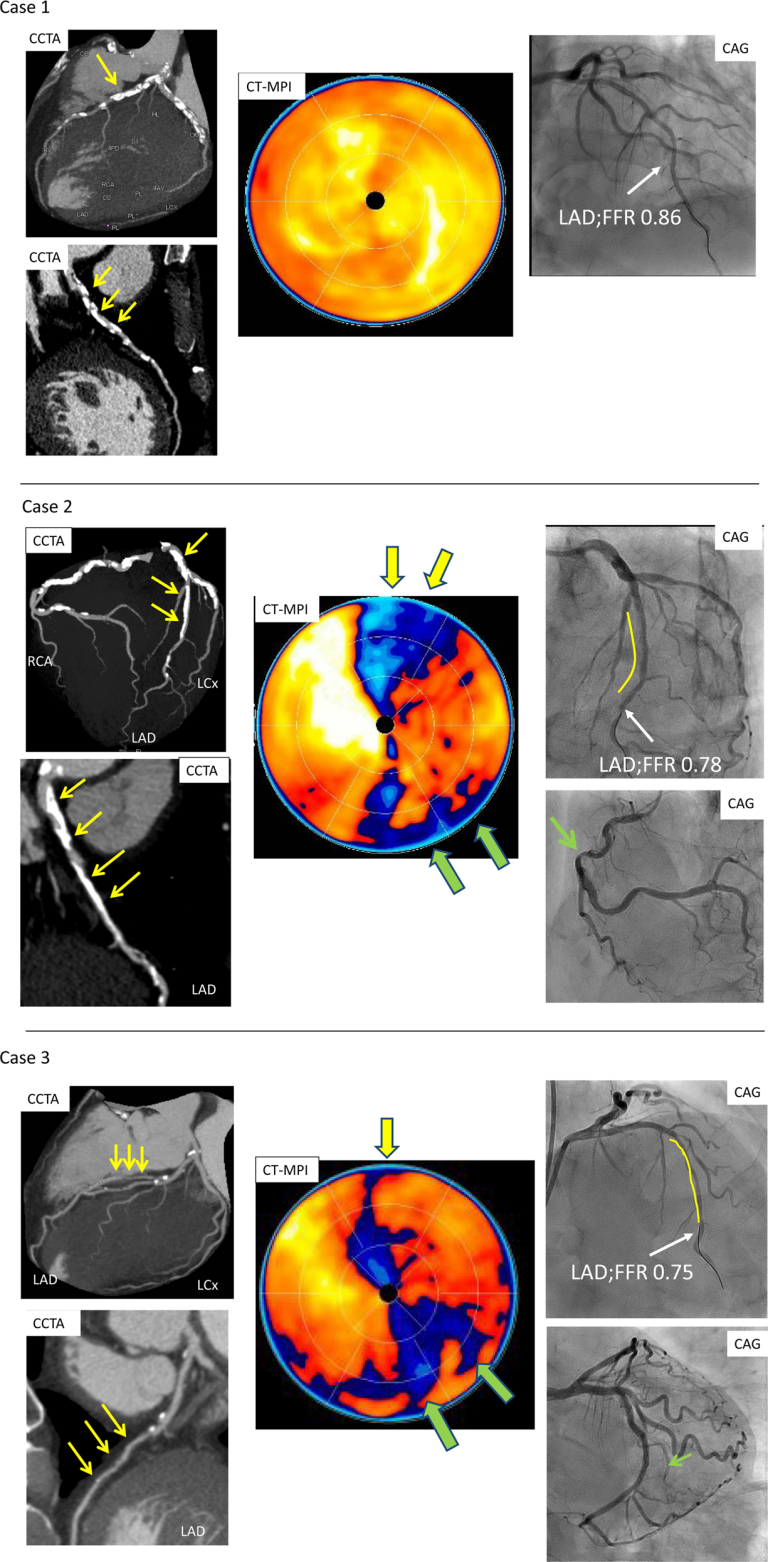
Case examples of CCTA, CT-MPI without stress, coronary angiography, and invasive FFR. Case 1. CCTA showed significant coronary artery calcification in the proximal LAD (yellow arrow). CT-MPI without stress showed no perfusion defect. Coronary angiography confirmed no significant stenosis in the LAD with invasive FFR = 0.86. Case 2. CCTA showed significant coronary artery calcification in the left main trunk and proximal LAD (yellow arrow). CT-MPI without stress showed perfusion defects in the anterior (yellow arrow) and inferior (green arrow) walls. Coronary angiography confirmed 58% luminal stenosis in the LAD with invasive FFR = 0.78 (yellow line) and 56% stenosis in the RCA (green arrow). Case 3. CCTA showed diffuse stenosis <50% in the middle of the LAD (yellow arrow). CT-MPI without stress showed perfusion defects in the anterior (yellow arrow) and inferolateral (green arrow) walls. Coronary angiography confirmed diffuse 55% stenosis in the LAD with invasive FFR = 0.75 (yellow line) and 69% of luminal stenosis in the LCx. LCx, left circumflex artery; RCA, right coronary artery.
